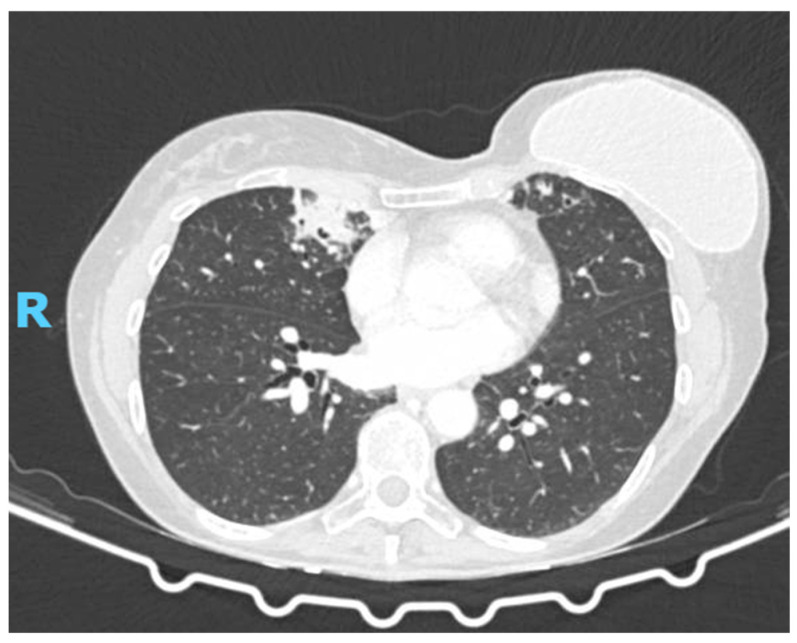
The chest CT scan revealed new pseudonodular opacities in the middle lobe.
